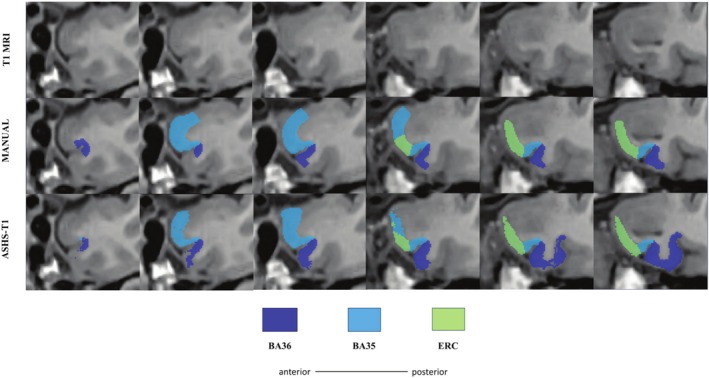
Comparison of manual and automatic segmentation (ASHS‐T1) on one of the cases from the in vivo dataset. The MRI slices shown approximately correspond to the six slices in the anterior MTL indicated in Figure 2. Some differences between the two approaches can be observed, for example for BA36. Note that the differences observed here may not be the same for all cases. Abbreviations: BA, Brodmann area; ERC, entorhinal cortex.
